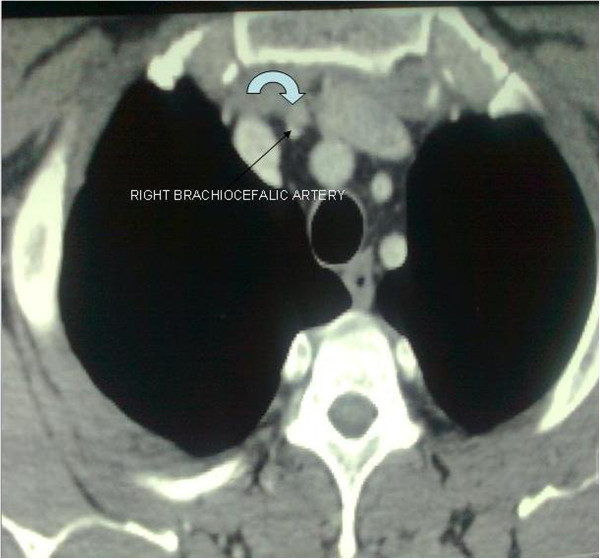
Chest CT of a cervical intrathoracic nodule. Chest CT revealing a cervical intrathoracic nodule, about 1 cm in diameter, in close contact with the thymus gland.
